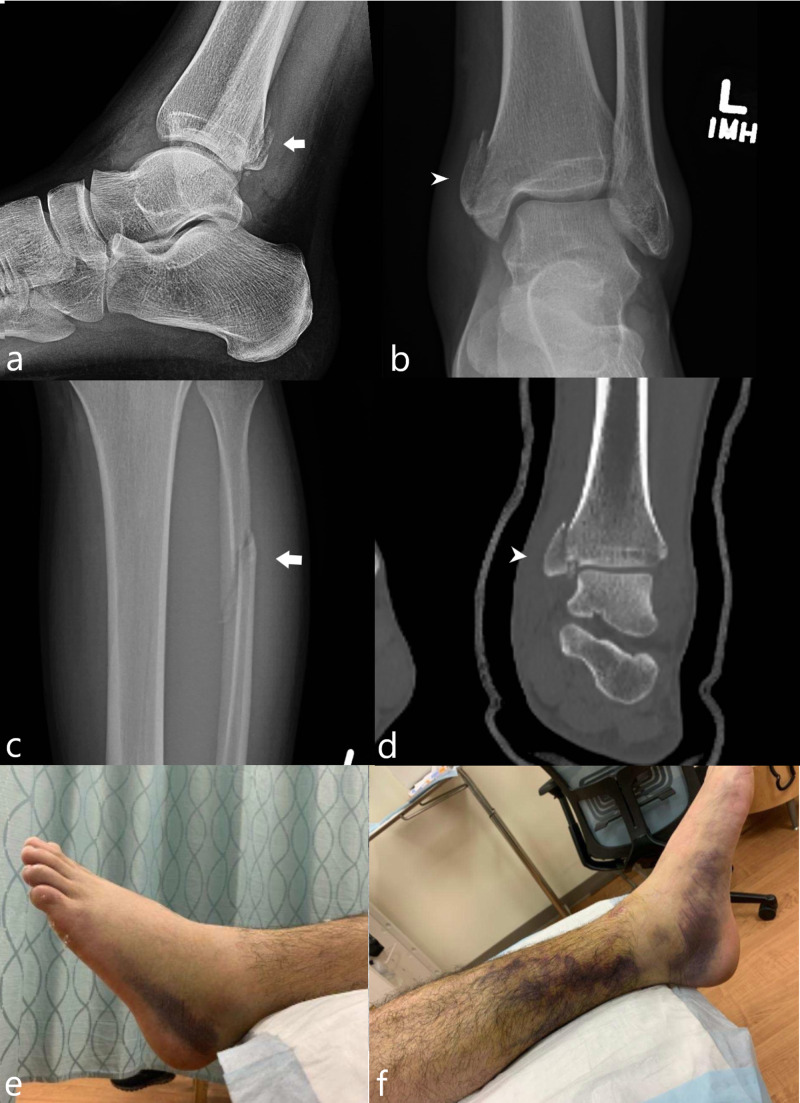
Case 4 radiologic and medical photos A lateral radiograph of the ankle (a) demonstrates a mildly displaced posterior malleolar fracture (white arrow). A frontal radiograph (b) demonstrates a mildly displaced fracture of the medial malleolus (white arrowhead).  A frontal radiograph of the proximal tibia (c) demonstrates an oblique fracture of the proximal fibular diaphysis (white arrow). A coronal computed tomography (CT) image (d) demonstrates mild comminution of the medial malleolar fracture (white arrowhead). Pictures taken after the patient sustained the landing injury (e, f) demonstrate swelling and ecchymosis.
